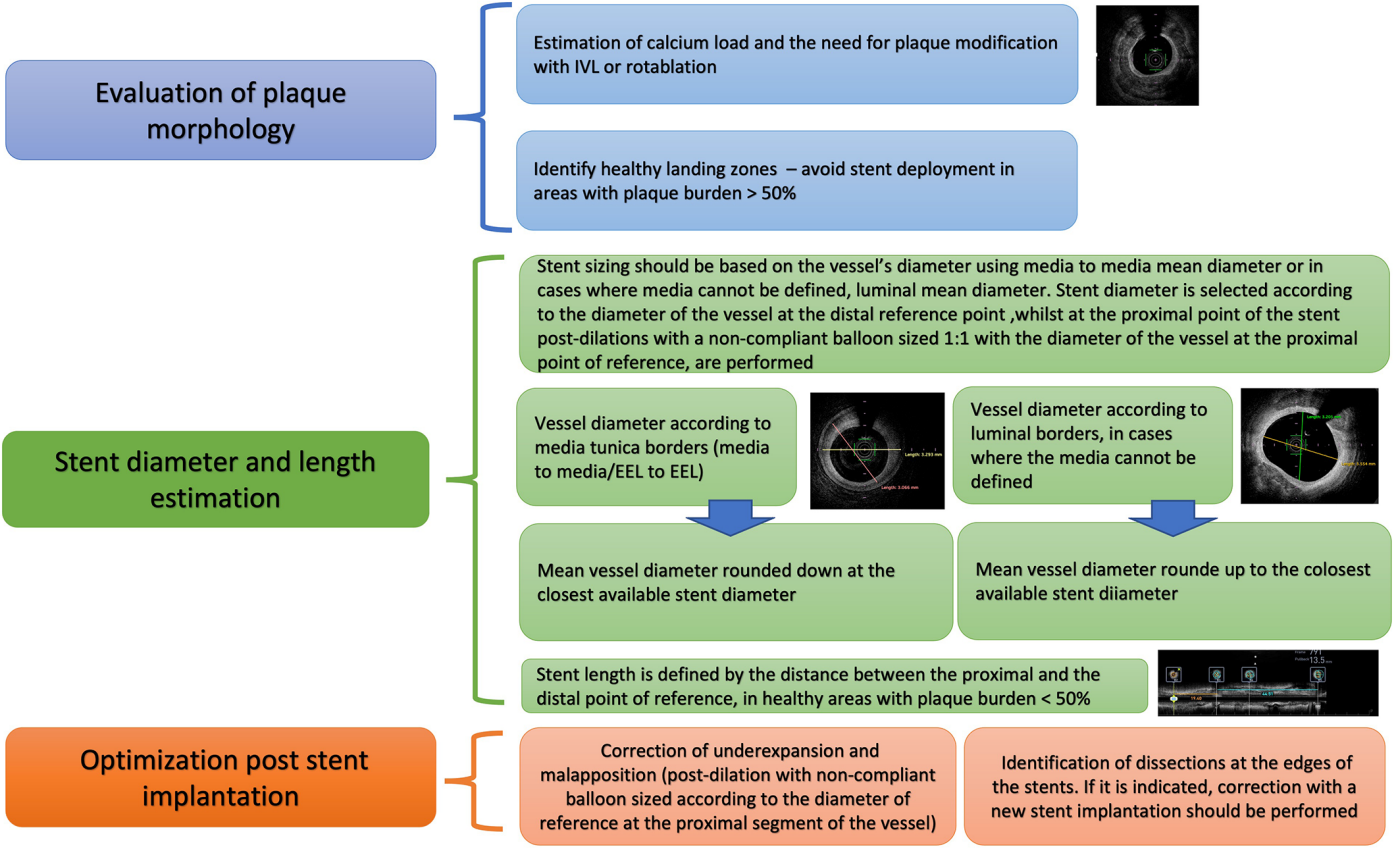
Proposed algorithm for intravascular imaging guided CTO PCI: precise evaluation of plaque characteristics and proper guidance for plaque modification, estimation of stent diameter and length and stent optimization post stent implantation. IVUS, Intravascular ultrasound; CTO, Chronic total occlusions; PCI, Percutaneous Coronary Intervention; EEL, external elastic lamina.
